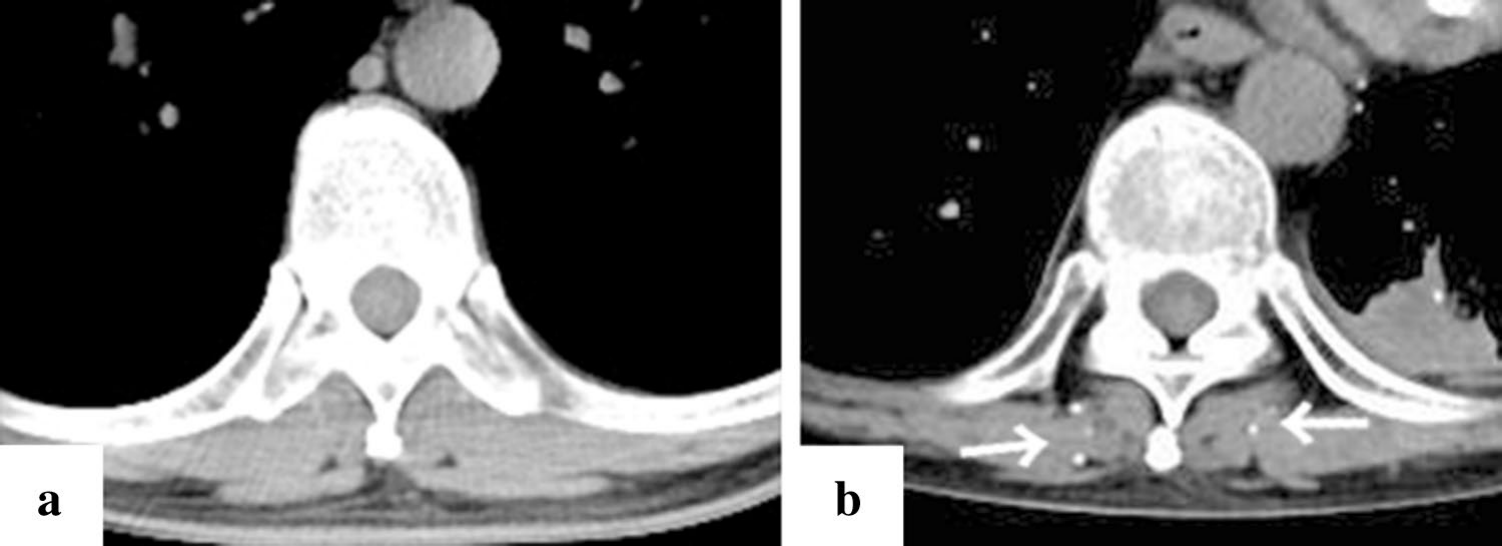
Representative case of skeletal muscle calcification in a 41-year-old patient (case no. 12). Computed tomography (CT) imaging on day 3 since the onset of symptoms (a) and after one month of the admission (b). CT imaging on admission showed no abnormalities in the skeletal muscle (a), and CT imaging one month after admission showed calcification in the skeletal muscle (b, white arrow)
